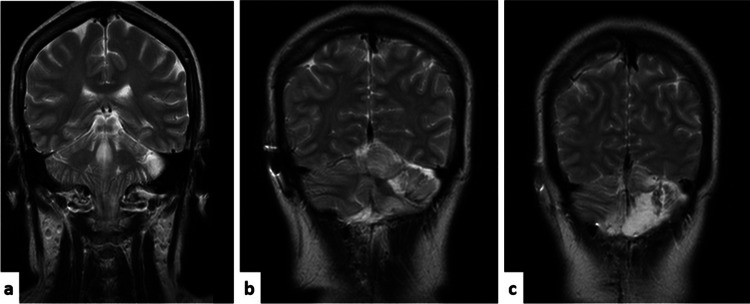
Coronal MRI with a cerebellar left hemispheric lesion hyper-intense in T2 signal, with thinning of white matter (hyperintense) and thickening of gray matter (isointense) with a striped appearance. This is an original figure.
